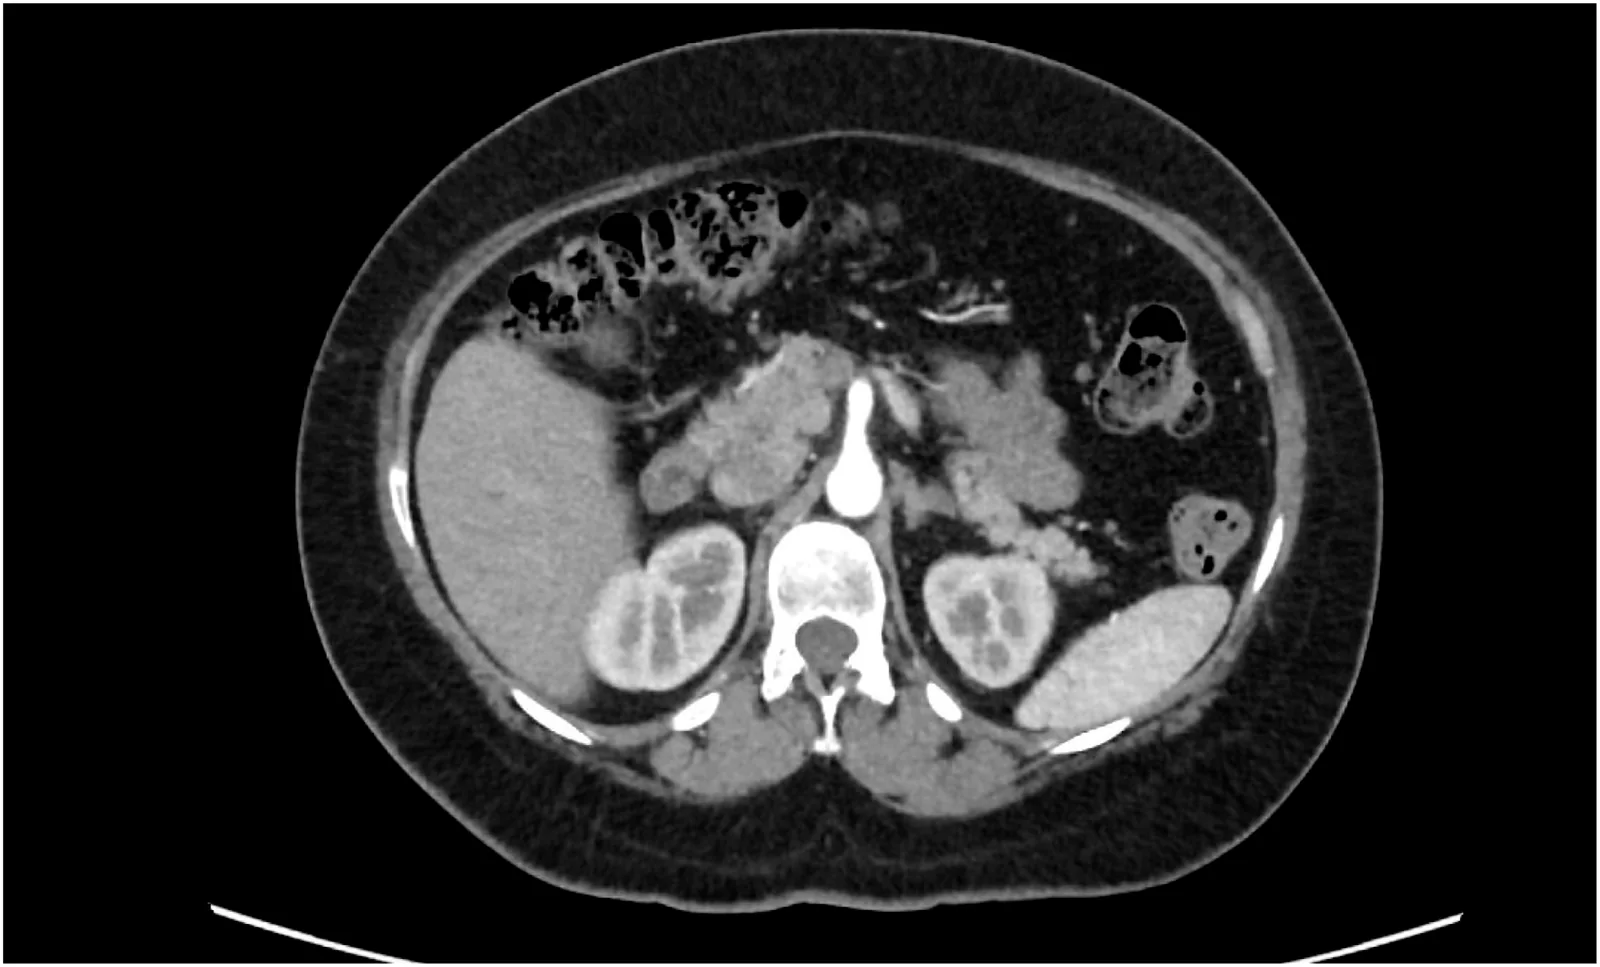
Abdominal CT scan showing two left adrenal lesions (arrows) consistent with adrenal adenomas. Representing CT-abdomen showing two left adrenal lesion seen show features keeping with adrenal adenomas.
